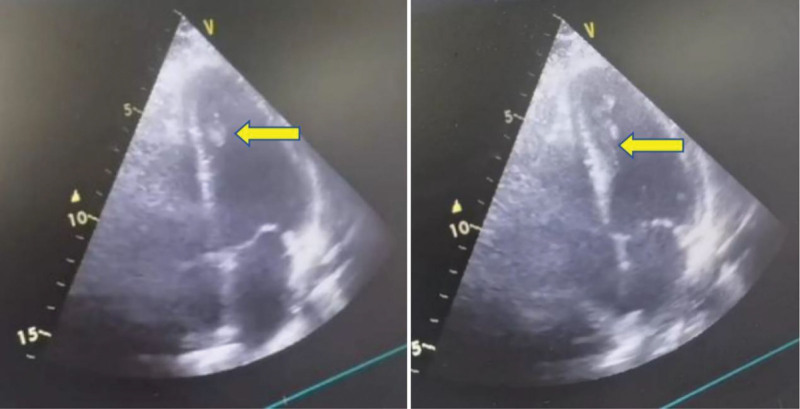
Bedside echocardiography revealing a left ventricular thrombus (yellow arrow).
